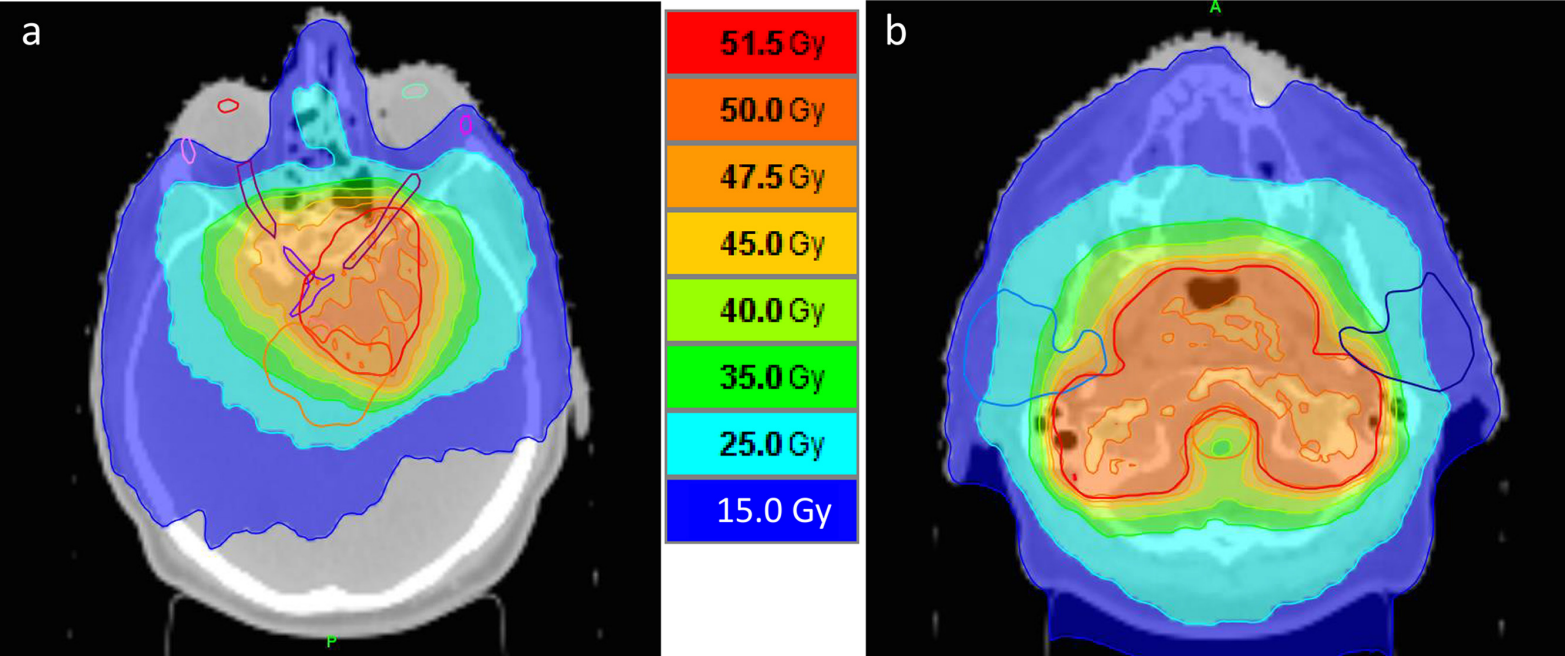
Axial CT scan showing intensity-modulated radiotherapy dose plan. The radiotherapy dose was 50 Gy in 30 fractions over 6 weeks. intensity-modulated radiotherapy was delivered with helical TomoTherapy, using daily image guidance and positional correction.^4^ The planning target volume is shown in red. In (a) the lenses, lacrimal glands, optic nerves and chiasm and brain stem are contoured; in (b) contours for the parotid glands and brain stem are shown. Planning organ at risk volumes for the optic structures and brain stem were created, but are not shown.
